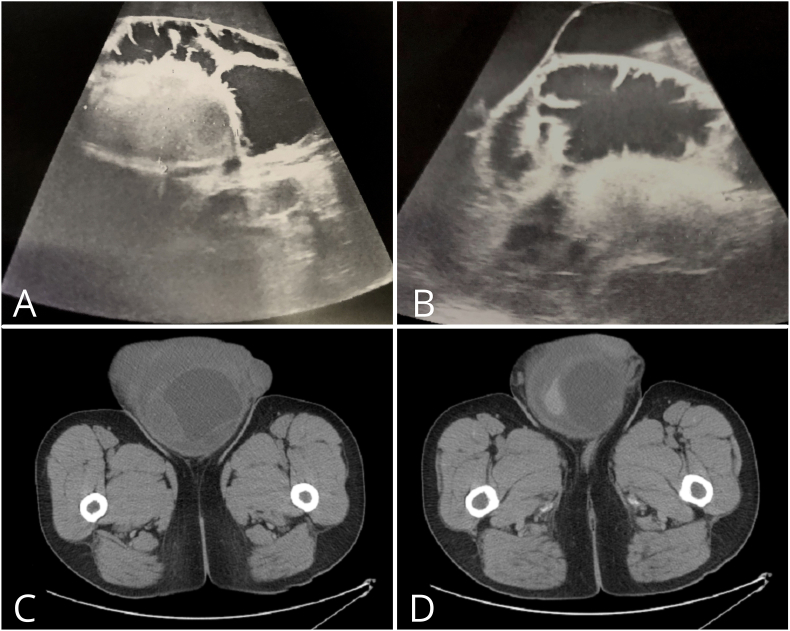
Ultrasonography of the scrotum, visible heterogeneous echotexture of the right testis and fluid collections (A). Ultrasonography of the scrotum, visible left testis and fluid collections (B). Contrast-enhanced CT scan, visible heterogeneous mixed solid and cystic mass of the right testicle (C,D).
